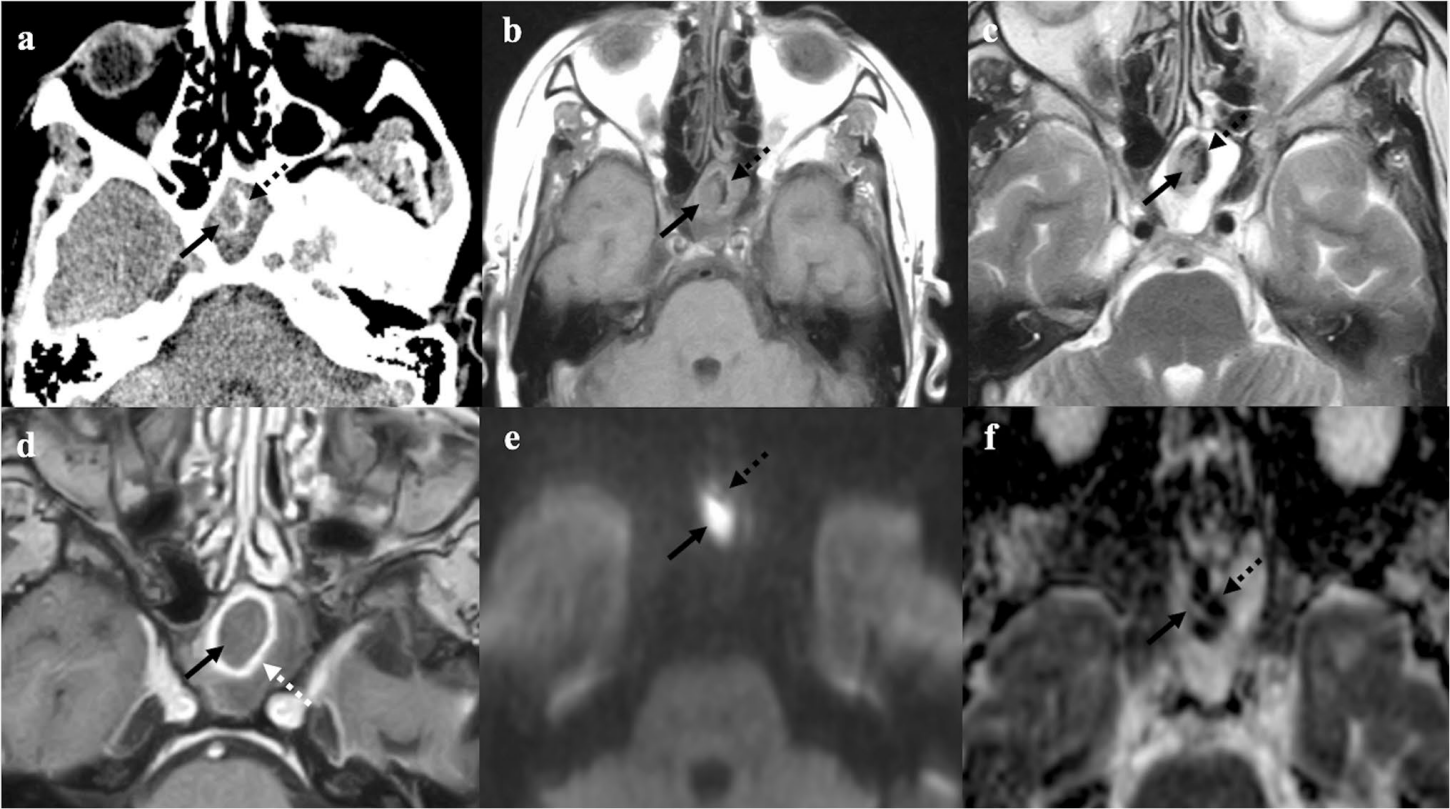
Fungus ball of the left sphenoid sinus (black arrows) in a 61-year-old female patient complaining of headache. CT shows a soft tissue density mass within the left sphenoid sinus with peripheral foci of calcific deposit due to fungal hyphae (black dotted arrows). Complete sinus opacification indicates obstruction of the ipsilateral spheno-ethmoidal drainage recess (a). MRI shows a mass in a completely mucous-filled left sphenoid sinus: the lesion is characterised by intermediate-to-low T1W (b) and T2W (c) SI and intralesional calcified foci with very low SI (black dotted arrows) similar to the air signal. Peripheral rim enhancement is seen on the axial T1W image obtained after gadolinium contrast injection (white arrow, d). The fungus ball shows intralesional areas of low SI on b800 DWI trace (e), and very low ADC values (f) due to the presence of calcifications and paramagnetic metals of fungal hyphae (black dotted arrows). These findings are suggestive for non-invasive fungal infection
